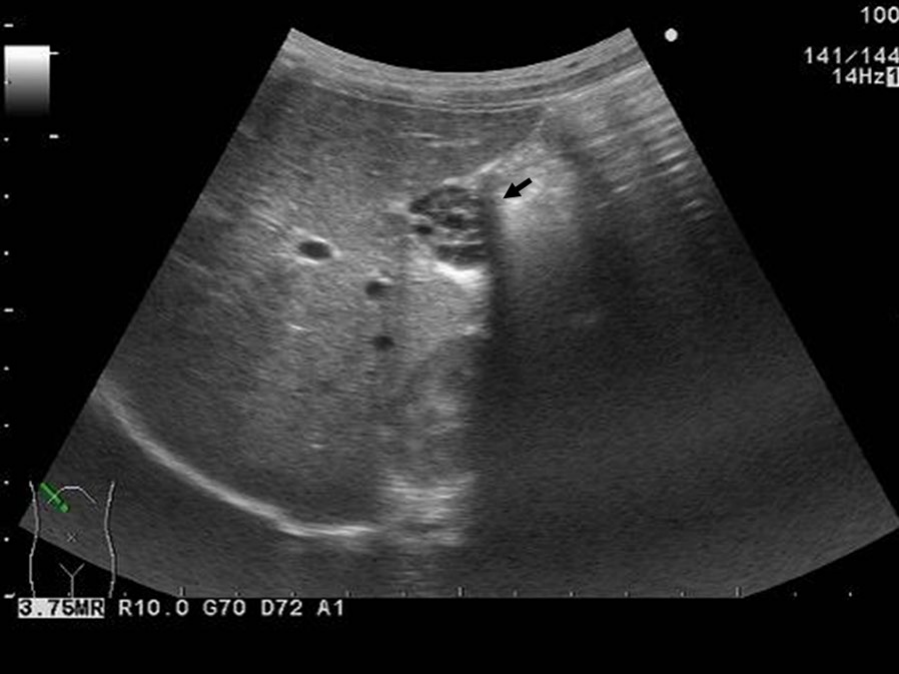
Abdominal ultrasonography. Abdominal ultrasonography showed multiple septa throughout the lumen of the gallbladder, giving it a honeycomb appearance (arrow). No gallstones or masses were identified
